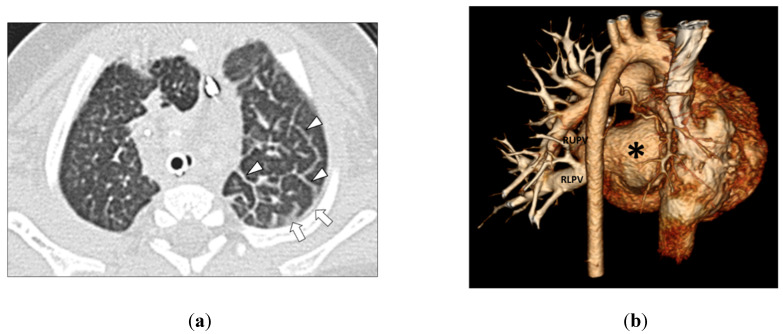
(a) 3-month-old girl with left-sided pulmonary vein stenosis but no history of aspiration. Axial lung window CT image shows left-sided septal thickening (arrowheads) and pleural thickening (arrows) in the left hemithorax. (b) 3-month-old girl with absent left pulmonary veins due to complete obstruction from severe pulmonary vein stenosis but no history of aspiration. The posterior view of the three-dimensional volume-rendered CT image of the vascular and heart strictures shows absent left pulmonary veins (asterisk). Normal and patent right upper pulmonary vein (RUPV) and right lower pulmonary vein (RLPV) are seen.
